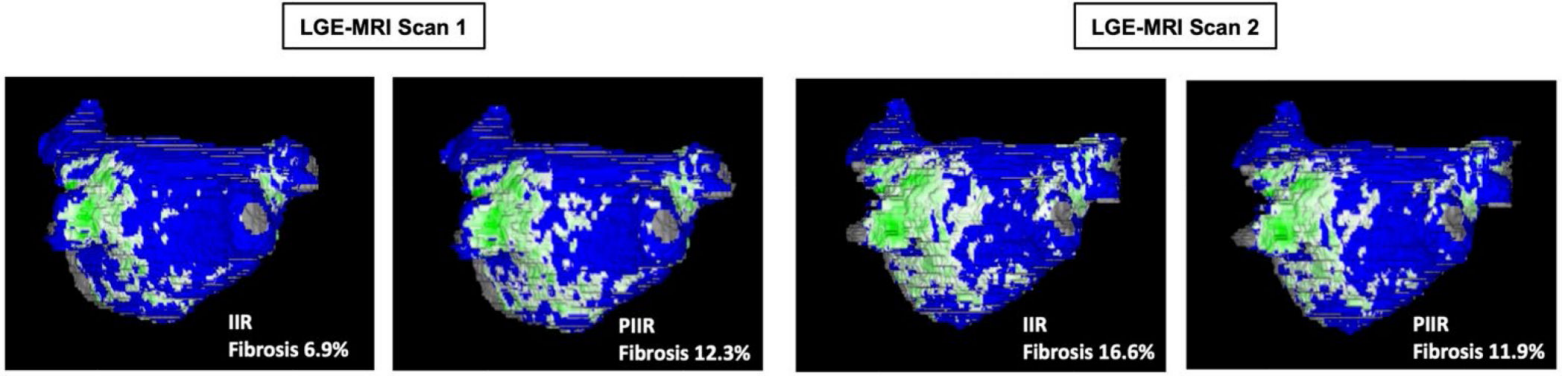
Reproducibility of LA fibrosis assessment using IIR and PIIR methods. The patient had two atrial 3D LGE scans during the same MRI session. Each scan was independently segmented by two experienced operators. IIR method with the widely used IIR threshold of 1.22 gave considerably different fibrosis values (6.9% and 16.6%) for scan 1 and 2. Whereas, the PIIR method gave similar fibrosis values (12.3% and 11.9%) for both scans. The PIIR threshold for scan 1 was 1.164 and the PIIR threshold for scan 2 was 1.263.
